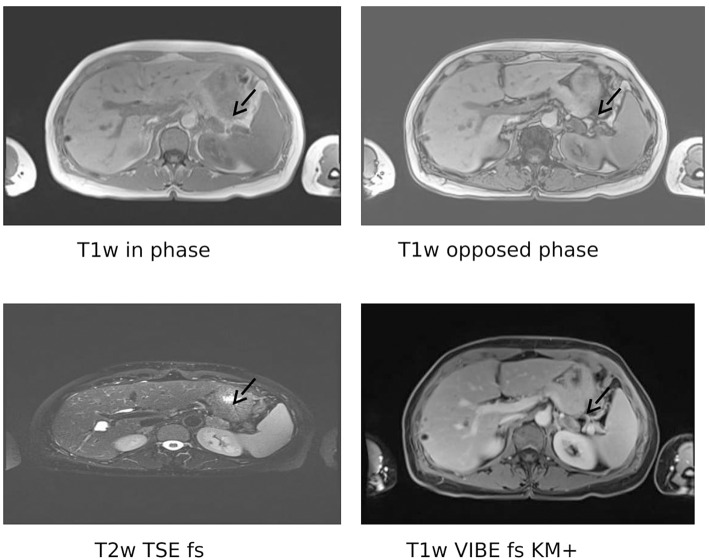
Magnetic resonance imaging. Shown is a left-sided adrenal mass (arrows) with a maximum diameter of 2.3 cm. Only slight signal loss in the opposed phase on Tl weighted-images. Mild contrast-enhancement in the periphery of the tumor. Multiple hepatic cysts. Tlw, T2w: Tl- or T2 weighted, respectively; TSE, turbo spin echo; VIBE, volumetric interpolated breath-hold examination; FS, fat saturated; KM+, with contrast agent.
